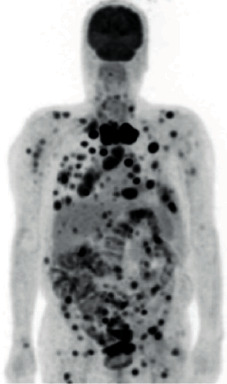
FDG-PET revealed multiple lesions with intense FDG uptake due to malignant disease (thyroid, mediastinal lymph nodes, lungs, bone, muscle, heart, liver, and adrenal).
